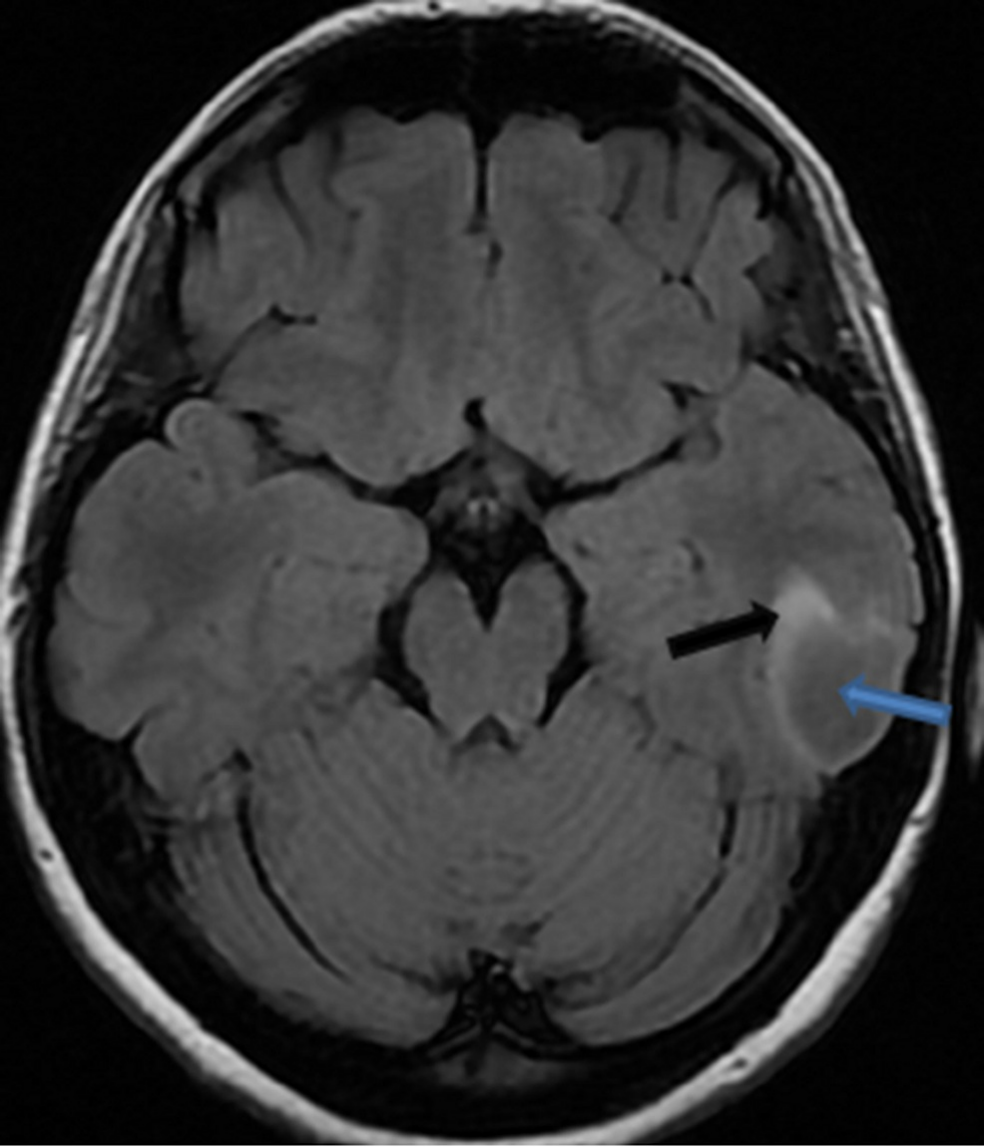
Axial FLAIR Left temporal cortical and subcortical lesion had incomplete suppression of the central aspect of the lesion (blue arrow) and a high-signal peripheral rim (black arrow).
